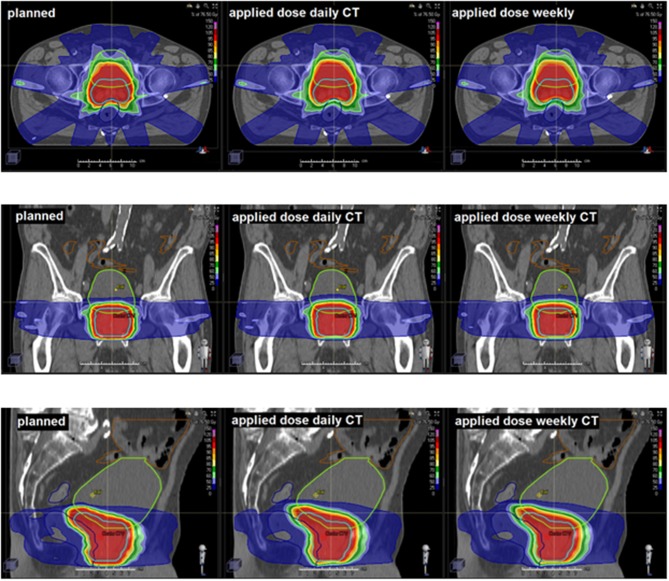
Representative CT slices demonstrating the planned and applied doses using daily or weekly CT-based repositioning.
